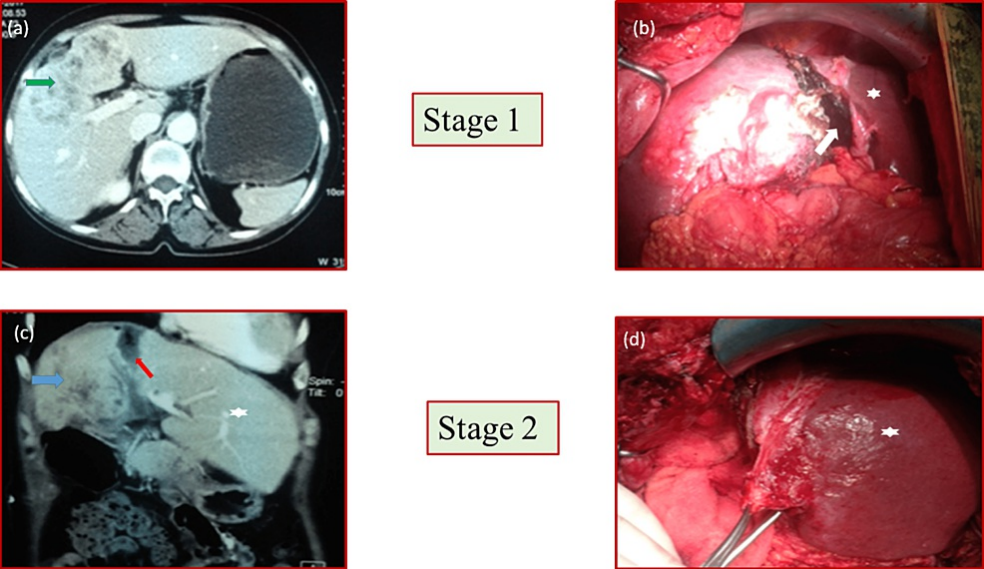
Two stages of partial ALPPS procedure (a) Preoperative CT scan of the abdomen showing a mass in segments IV and V of the liver (green arrow). (b) Partial transection of the liver (white arrow) with ligation of the right portal vein completing stage I. Remnant liver (left lateral sector) marked by the star. (c) CT scan after 10 days of Stage I showing hypertrophy of left lateral sector of the liver (marked by a star) with atrophy of right lobe with mass (blue arrow). The transection line is marked by the red arrow. (d) Completion of stage II with complete transection of liver and Roux en y hepaticojejunostomy. Significant hypertrophy of the remnant can be appreciated compared to (b) marked by the star. ALPPS: associating liver partition and portal vein ligation for staged hepatectomy
